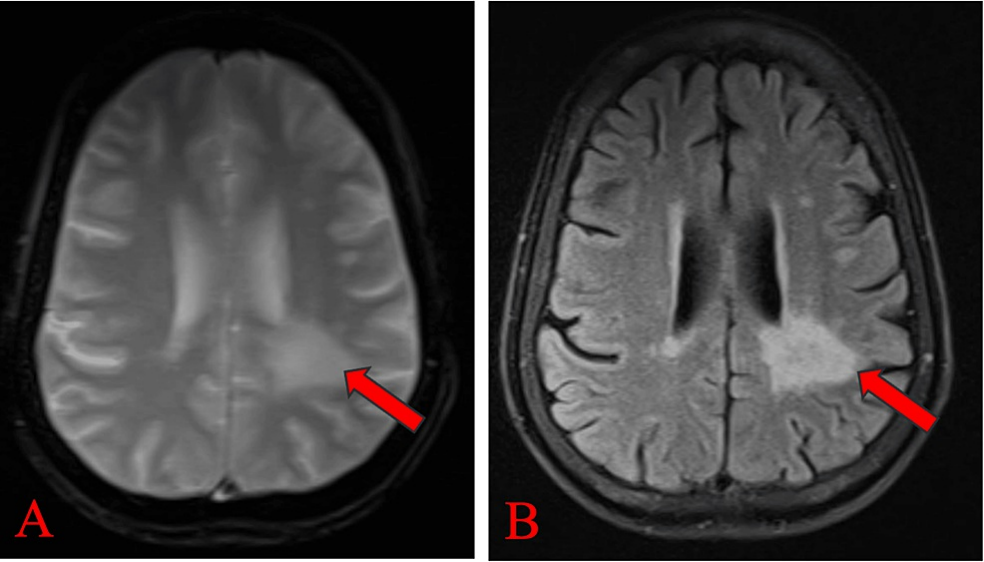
MRI brain (P+C) showing lesion in the left peritrigonal white matter extending in the left parietal white matter, which appears hyperintense on FLAIR (A), shows no blooming on GRE (B), as shown by red arrows. MRI brain (P+C): Magnetic resonance imaging brain plain and contrast; FLAIR: fluid-attenuated inversion recovery; GRE: gradient echo
